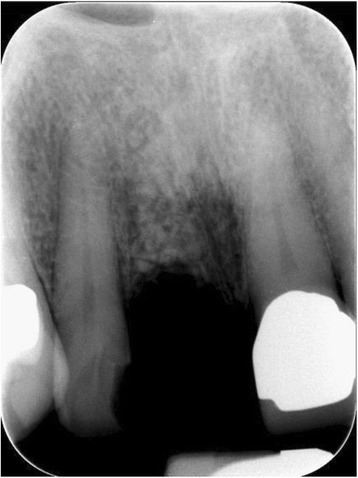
Single tooth X-ray immediately after the augmentation using autogenous dentin
